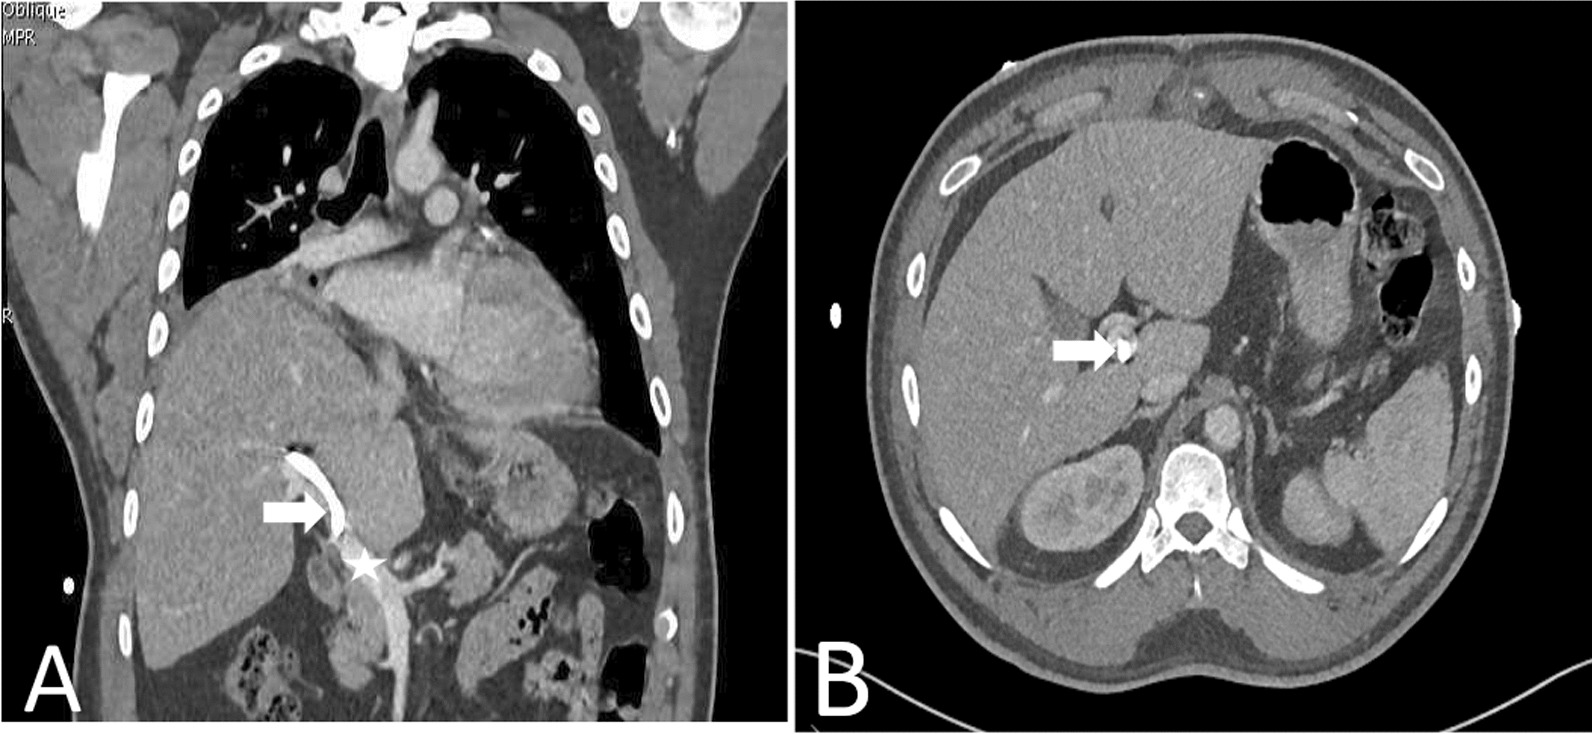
Coronal (A) and axial (B) CT images of the chest and abdomen with contrast enhancement reveal the presence of a right-sided pleural drainage catheter (white arrow) traversing through the right 7th intercostal space and penetrating the liver parenchyma, with the catheter tip positioned within the main portal vein (Star)
